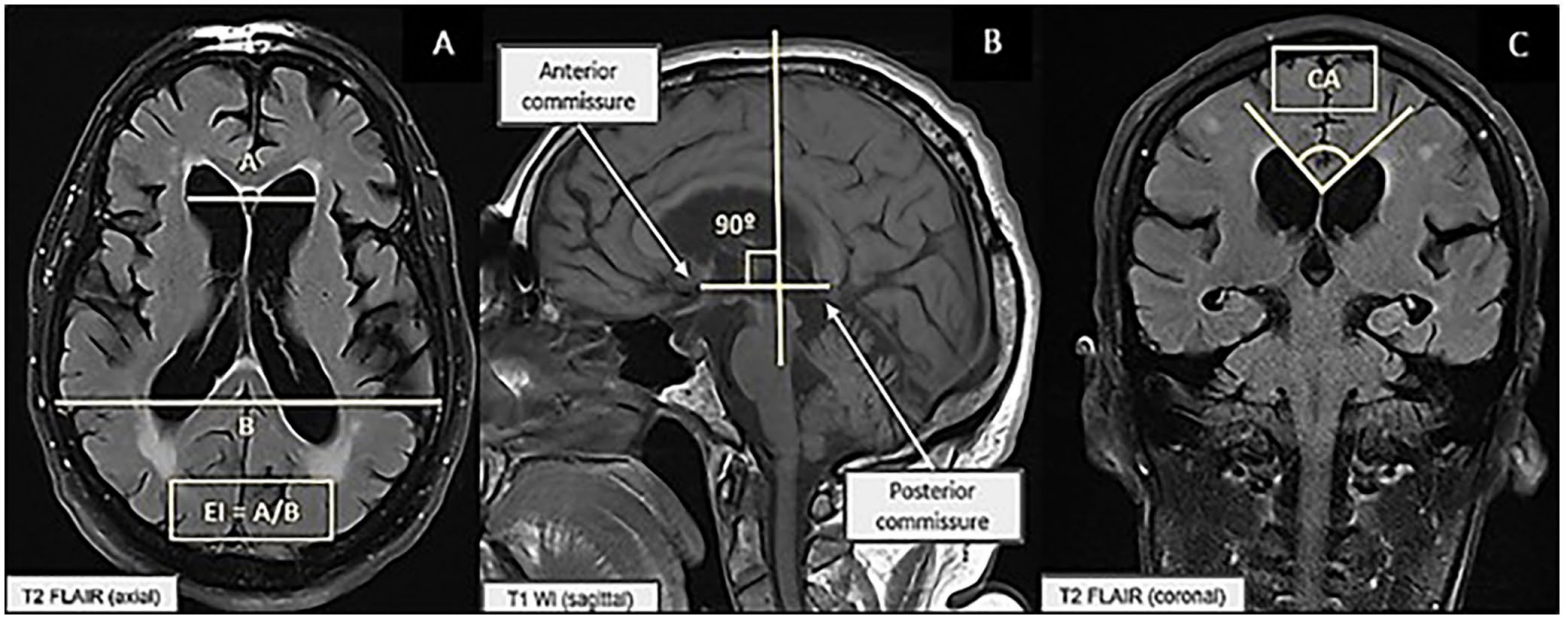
(A) Measurement of the Evans index. (B) Measurement of the callosal angle on sagittal T2 FLAIR (Fluid-Attenuated Inversion Recovery) brain MRI. (C) Measurement of the callosal angle on coronal T1-weighted brain MRI.
